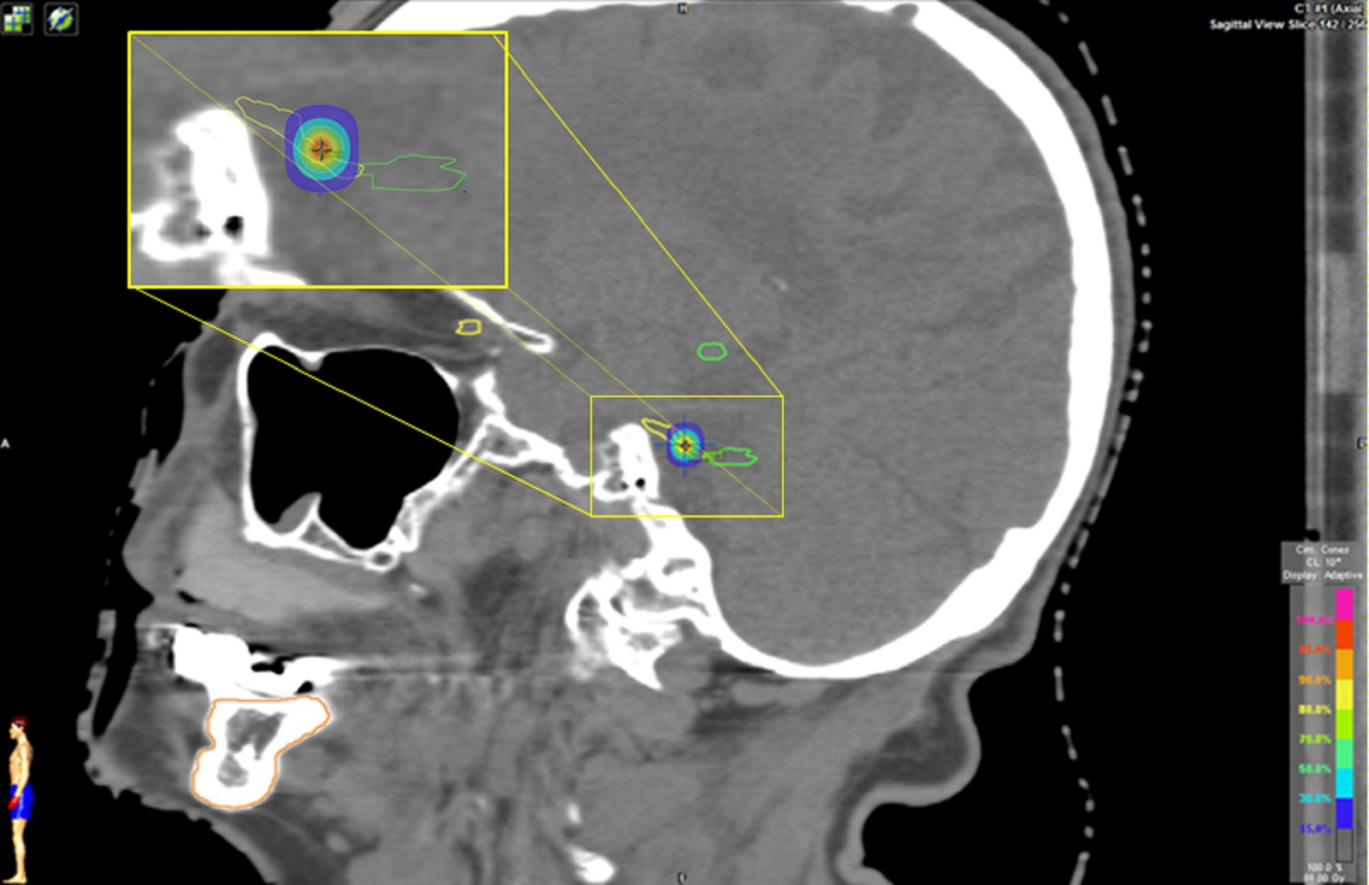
Sagittal Head CT. The image demonstrates targeted trigeminal nerve (contoured yellow) near its root entry into the brainstem (contoured green).
